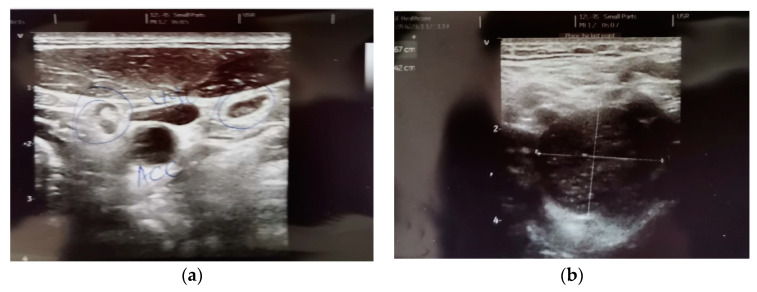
(a) Two nodular images, with the hyperechoic center of 0.66 and, respectively, 0.53 cm, between external to common carotid artery (CCA) and internal jugular vein (IJV). (b) Hypoechoic nodular image, with Doppler signal in the hilum, the transverse diameter of 2.57/2.42 cm; longitudinal diameter of 4.54/2.14 cm, between internal carotid artery (ICA) and external carotid artery (ECA), in direct contact with the vessels.—April 2021.
